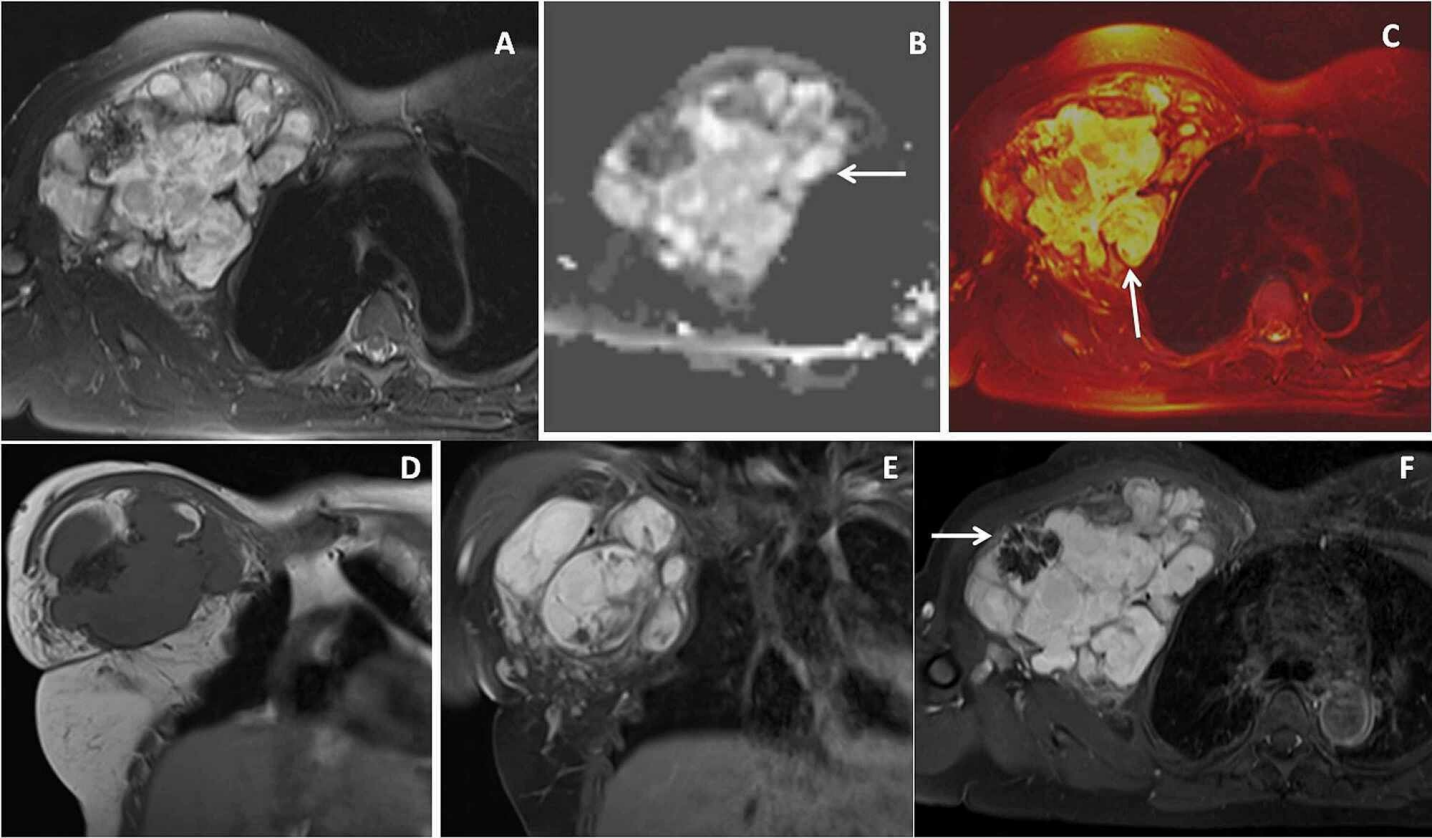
Chest wall malignant fibrous histiocytoma Thirty-six-year-old female with malignant fibrous histiocytoma (MFH) of right chest wall. Axial proton density fat-suppressed (PDFS) image (A) showed a larger lobulated heterogeneously hyperintense mass lesion in the right upper anterior chest wall causes anterior displacement of the pectoralis muscle. Axial diffusion-weighted image (DWI) (B) image shows bright signals within the mass (← arrow) while fusion image (C) between axial PDFS and DWI showed the bright signals in major portions of lesion (↑arrow). Coronal T1 weighted image (T1WI) (D) shows T1WI isointense signal intensities of lesion with ill-defined T1W hypointensities within. Fat-suppressed post gadolinium coronal and axial T1W images (E & F) showed heterogeneous enhancement of the lesion with non-enhancing T1 low signal intensity lesion(→arrow).
